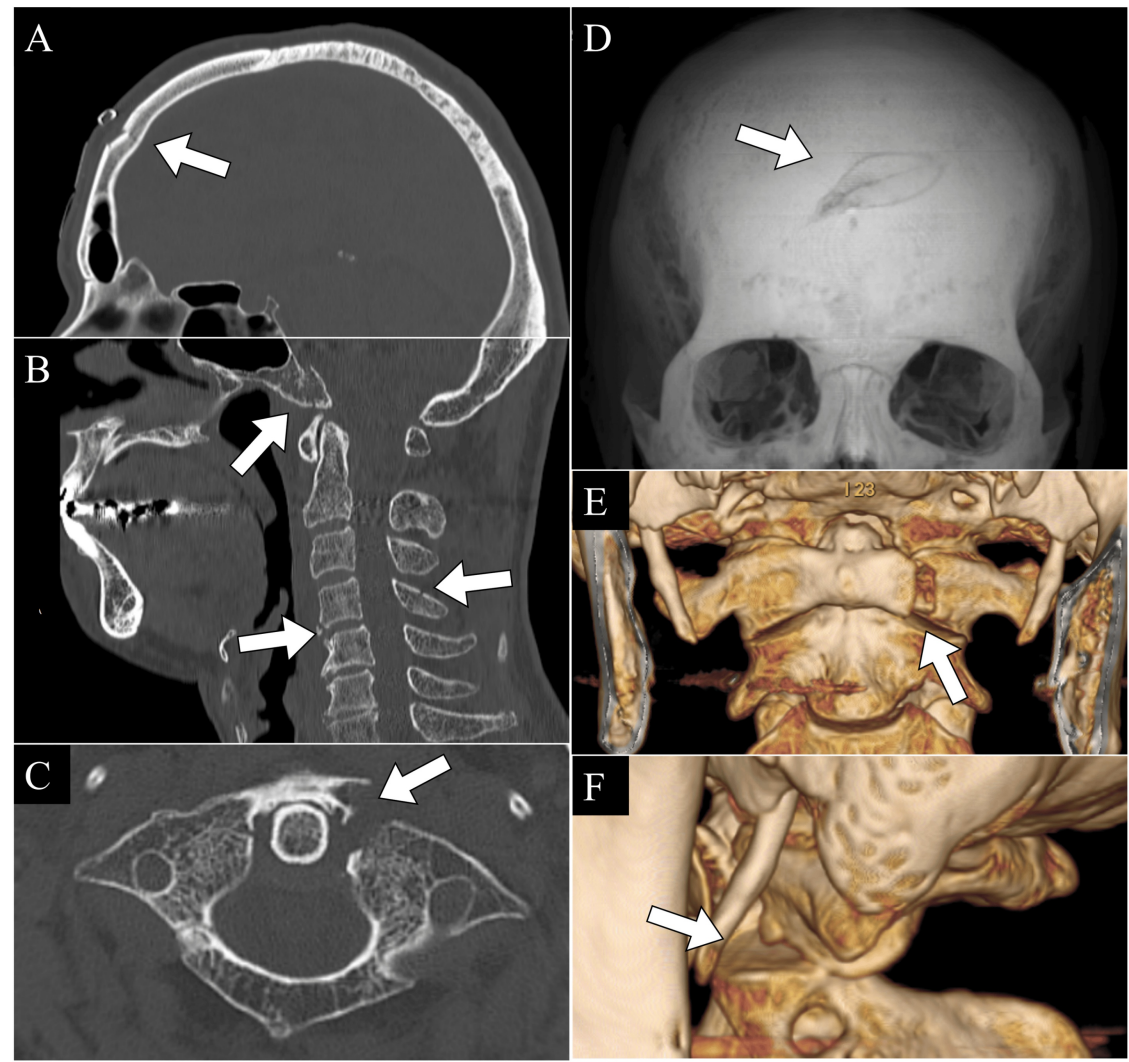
Head and cervical spine CT findings on the day following the injury (day X+1). A: Sagittal cut of the head CT, showing a fracture of the frontal bone. B: Sagittal cut of the cervical spine CT, revealing fractures of the occipital base, the vertebral body of C4, and the spinous process of C4. C: Axial cut of the cervical spine CT at the level of the atlas (C1), demonstrating a fracture of the anterior arch of the atlas with displacement. D: Three-dimensional reconstruction of the head CT, showing a fracture of the frontal bone. E: Three-dimensional reconstruction of the cervical spine CT, showing a fracture of the anterior arch of the atlas. F: Three-dimensional reconstruction of the cervical spine CT, revealing joint incongruity between the C1 and C2 vertebra. White arrows in A–F indicate the respective fracture sites.
